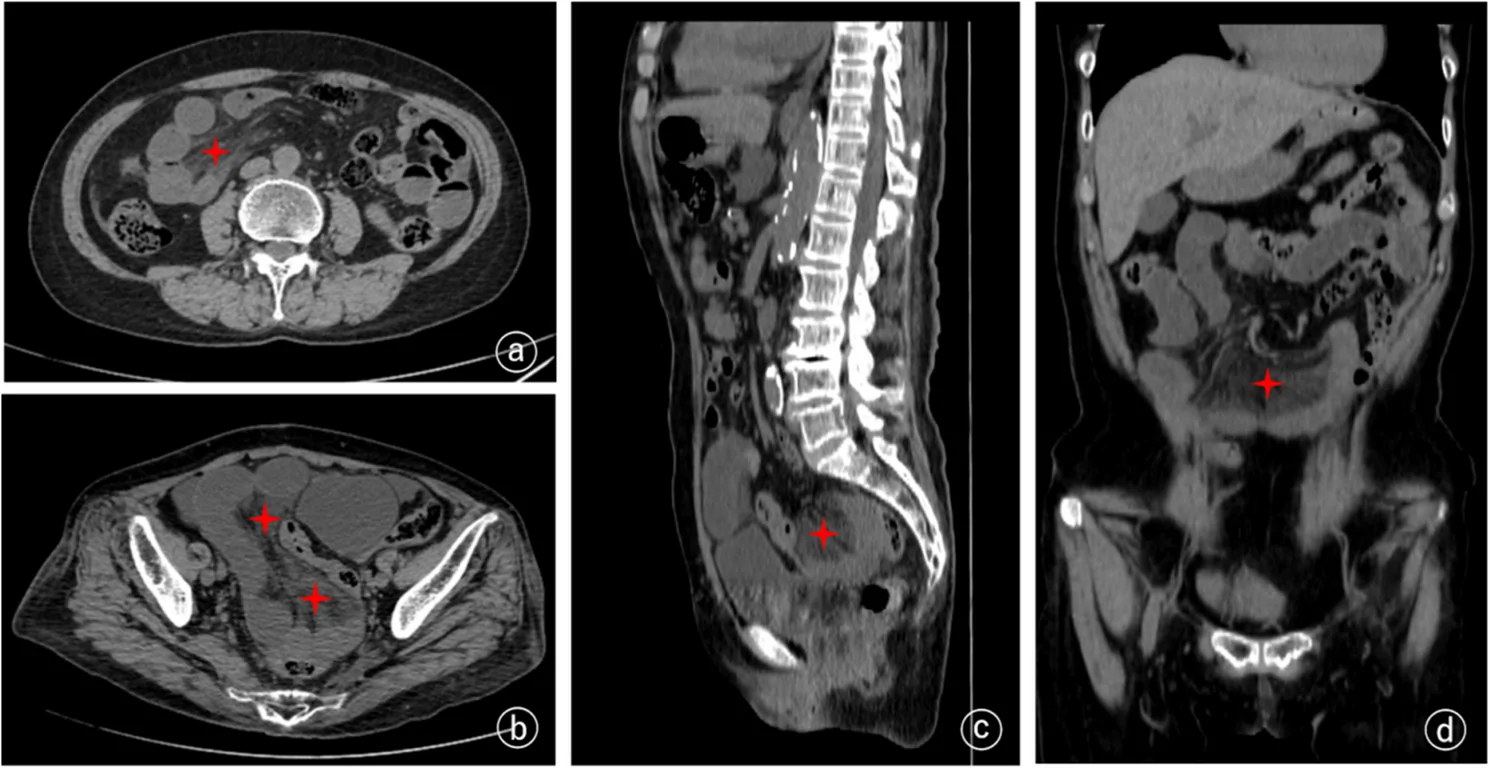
CT manifestations of mesentric edema red four-pointed stars: mesenteric edema; A, B Axial views; C sagittal view; D coronal view
